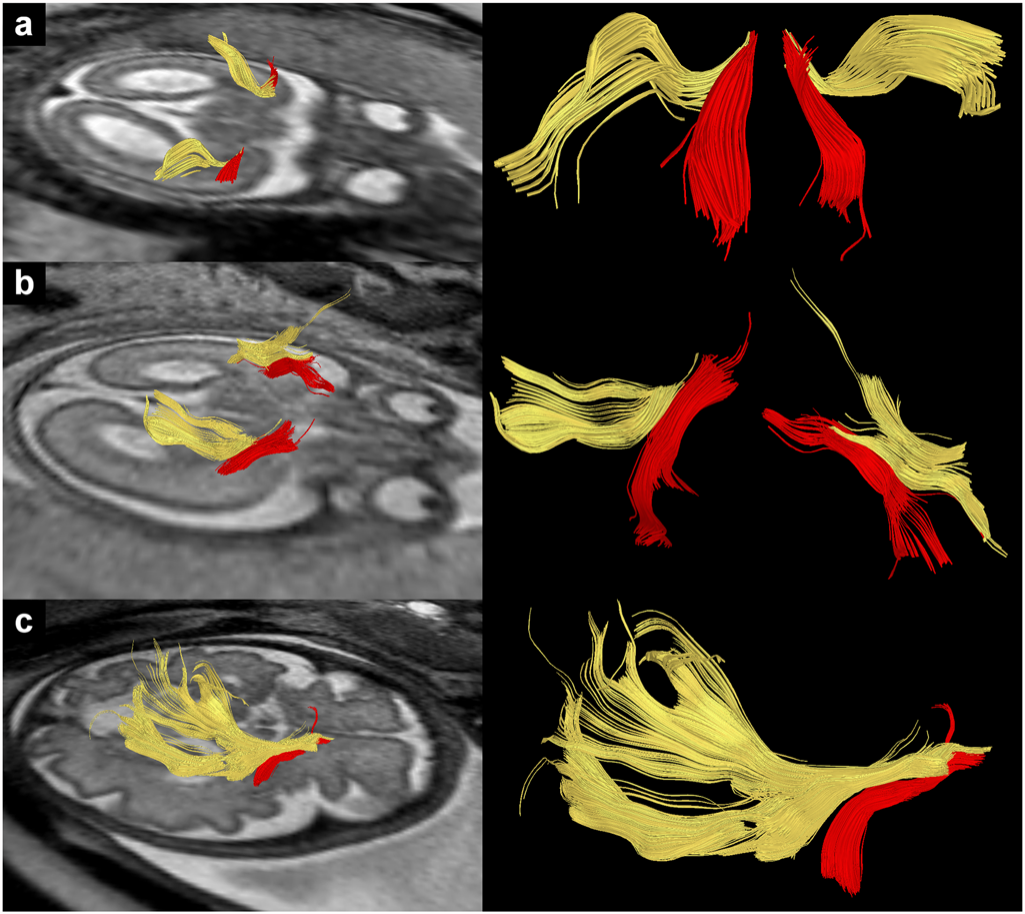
In utero tractography of the IFOF (yellow) in subjects 1 (a), 9 (b) and 23 (c) (20, 24 and 33 GW respectively) and its relation to the UF (red). Fiber tracts are projected on axial T2-weighted images.
